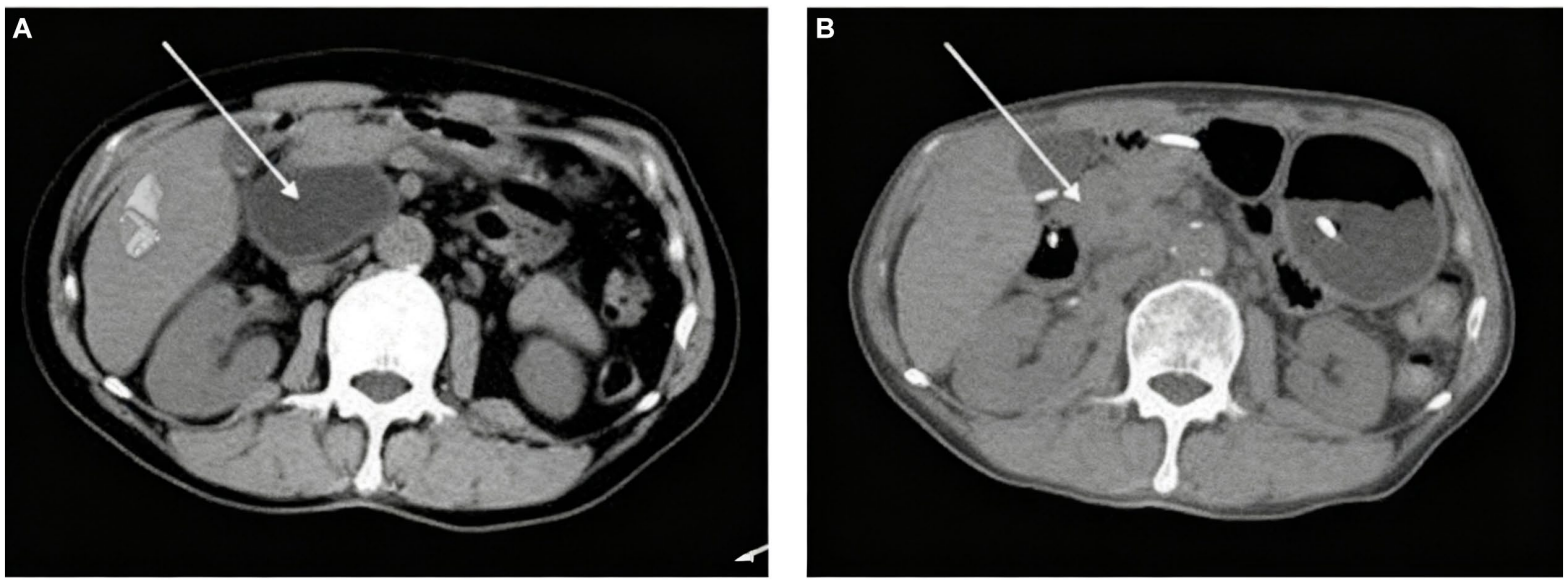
Abdominal CT scans demonstrate the (A) pancreatic enlargement with a cystic hypodence lesion posterior to the pancreatic head; (B) reduction in the size of pancreatic cystic lesion after 2 months.
